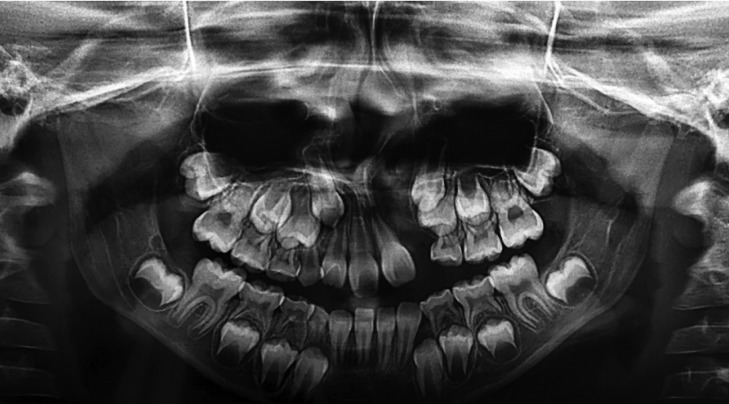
Panoramic radiograph showing agenesis of the left lateral incisor.
